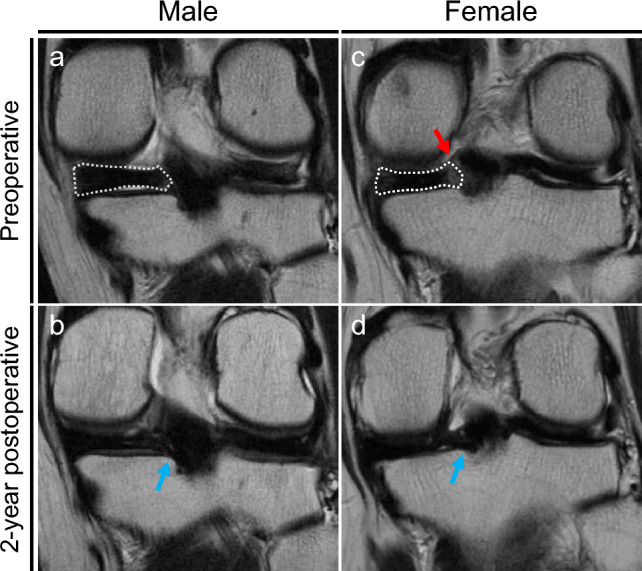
Representative magnetic resonance images (MRI) preoperatively and 2 years postoperatively in male (a and b) and female (c and d). a Preoperative coronal MRI presents Giraffe neck sign (dotted area). b Postoperative coronal MRI shows a continuity of the medial meniscus (blue arrow). c Preoperative coronal MRI presents Giraffe neck sign (dotted area) and cleft sign (red arrow). d Postoperative coronal MRI shows a continuity of the medial meniscus (blue arrow) (color figure online)
